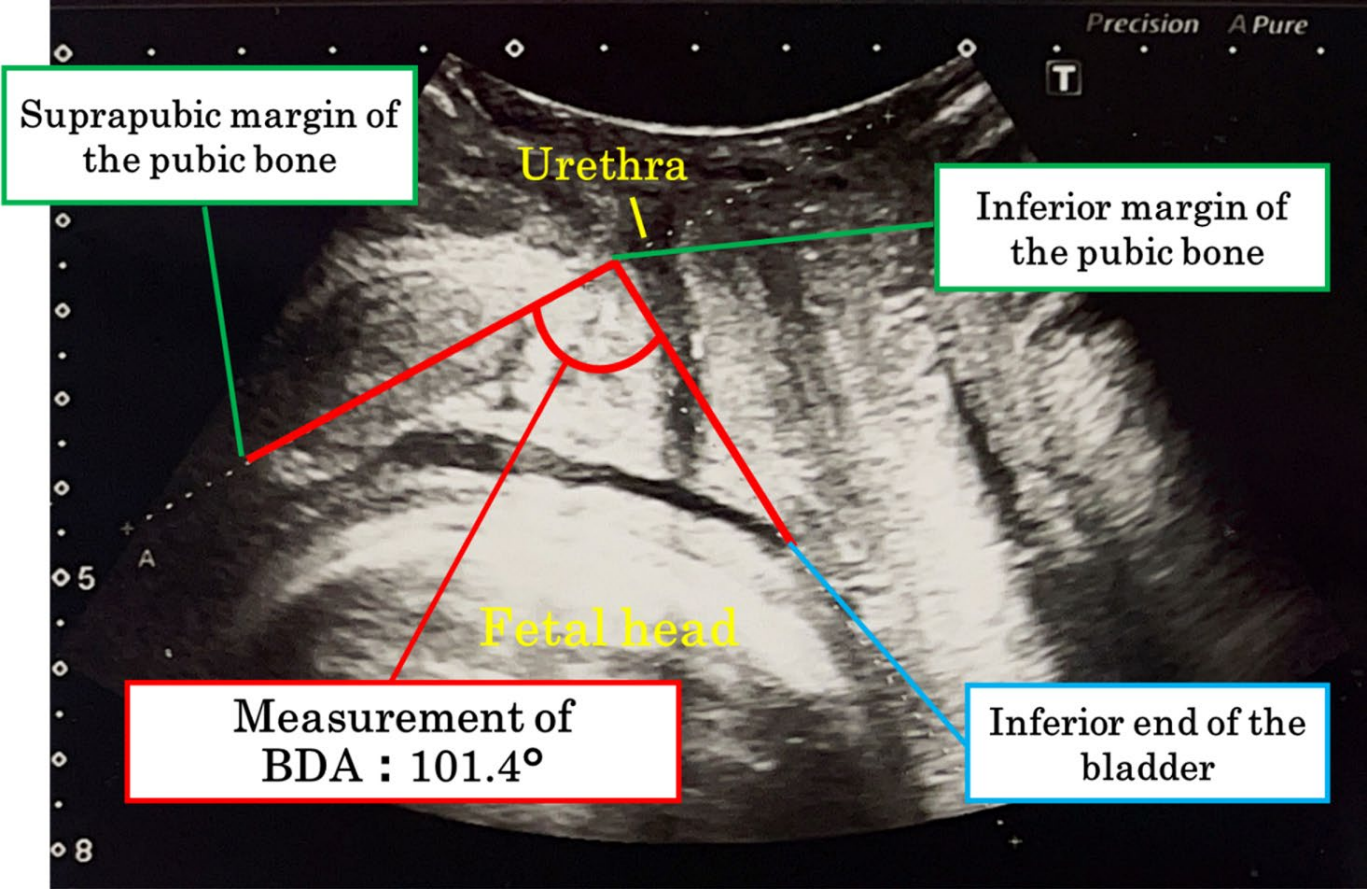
Measurement of the bladder descent angle (BDA). The BDA was defined as the angle between the long axis of the pubic bone and a line drawn from the inferior margin of the pubic bone to the inferior end of the bladder. This two‐dimensional ultrasonographic measurement provides a simple, reproducible, and objective marker of bladder displacement during late pregnancy.
